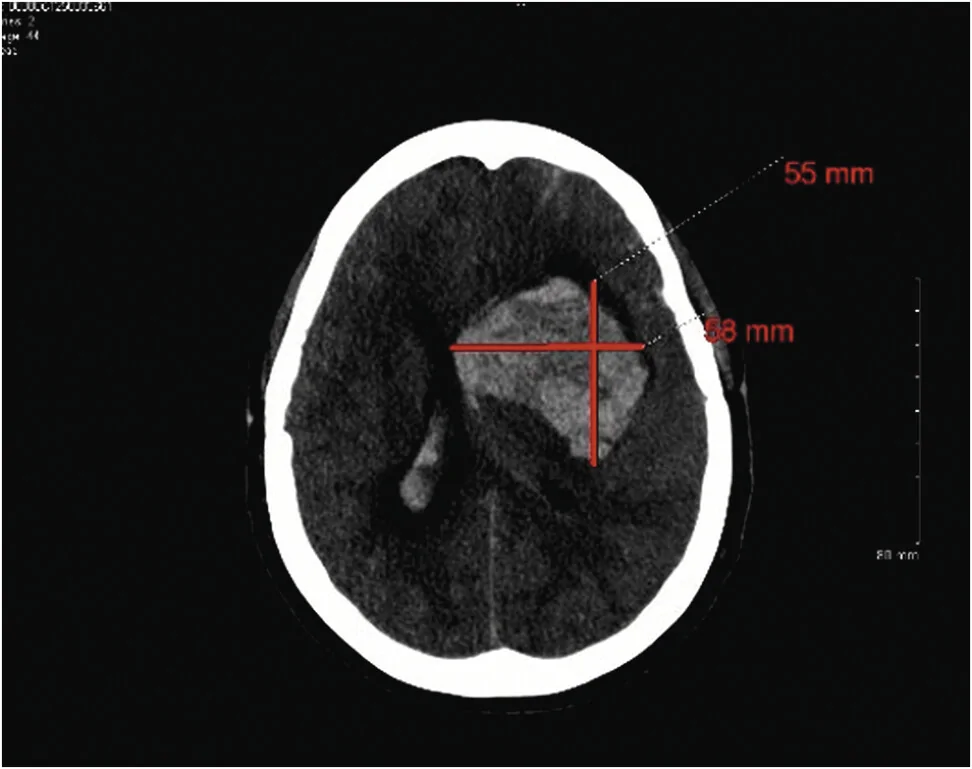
Computed tomography (CT) of the head in Case 2 demonstrating a large left intraparenchymal hemorrhage with surrounding edema, midline shift, and evidence of transtentorial herniation.
